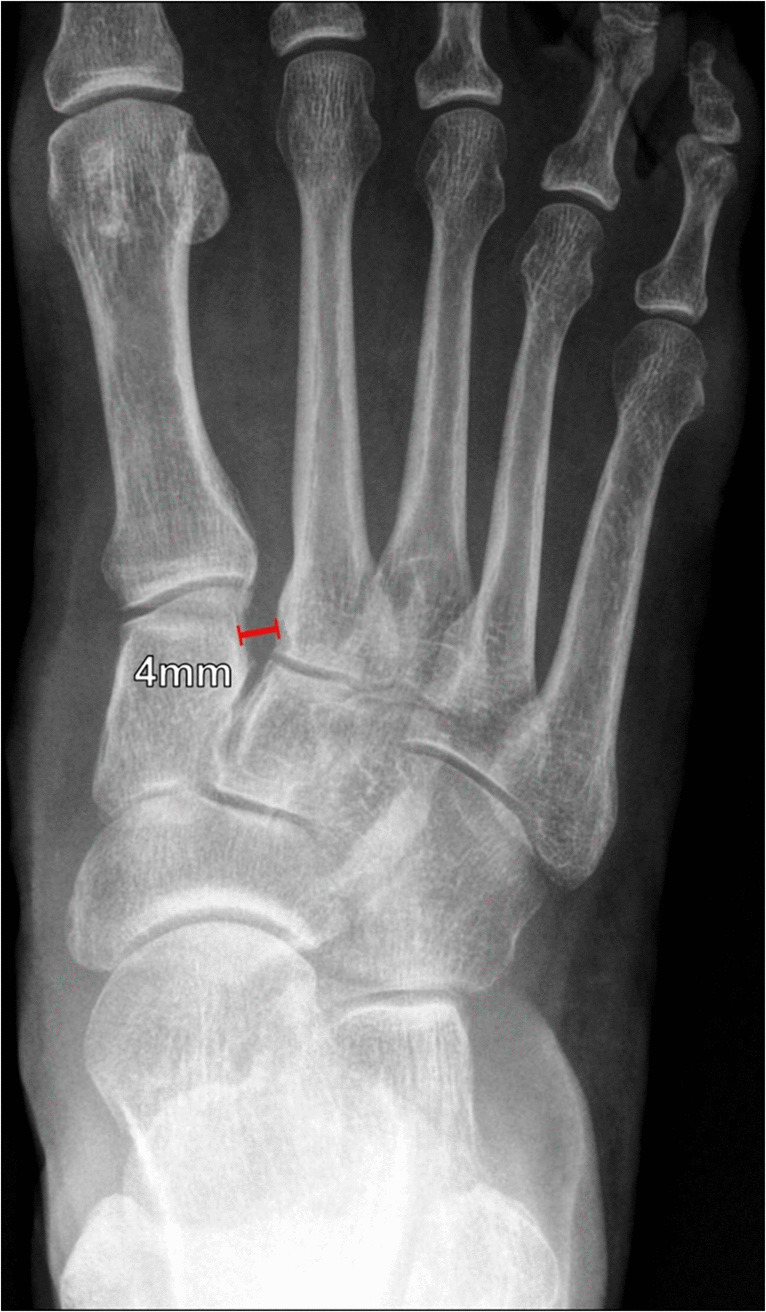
Lisfranc diastasis on DP weightbearing radiograph of the foot in a 21-year-old male football player following injury. Normally, the lateral margins of the medial cuneiform and the first metatarsal base are aligned, as are the medial margins of the middle cuneiform and second metatarsal base. The distance between the medial cuneiform and the second metatarsal base should measure ≤ 2 mm. A measurement > 2 mm or asymmetry compared with the asymptomatic contralateral foot suggests Lisfranc ligament insufficiency. In the imaging example, the space between the medial cuneiform and second metatarsal base measures more than 4 mm, indicating Lisfranc ligament complex insufficiency
